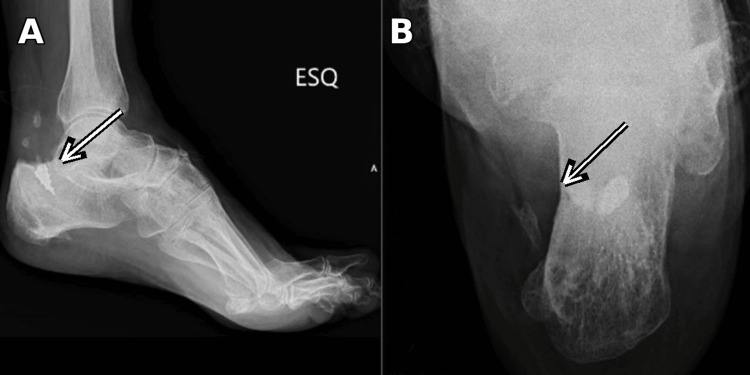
Two-year follow-up radiographs: lateral (A) and axial calcaneal (B) views demonstrating maintained tendon reinsertion with the suture anchors in place (arrows) and no degenerative change or calcaneal deformity.
